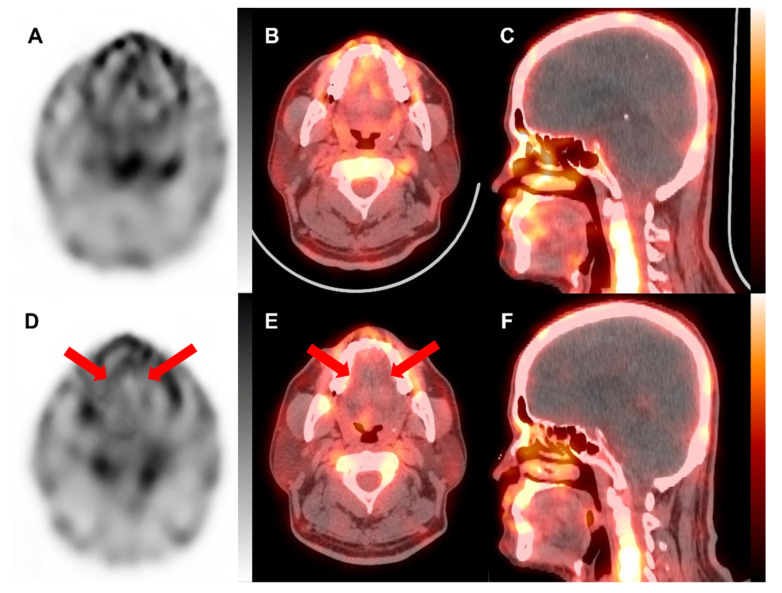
Transaxial single-photon emission computed tomography (SPECT), fused transaxial, and fused sagittal SPECT/computed tomography (CT) images of the tongue on the initial (A–C) and follow-up (D–F) blood pool SPECT/CT using Tc-99m methylene diphosphonate. On the initial blood pool SPECT/CT images, heterogeneously increased blood pool uptake was seen in the tongue. On the follow-up SPECT/CT images, blood pool uptake was prominently reduced over the entire area of the tongue (arrows on (D,E)). In the initial SPECT, the tongue showed unevenly increased intake in various areas, but in the follow-up SPECT, the tongue showed an overall decrease.
